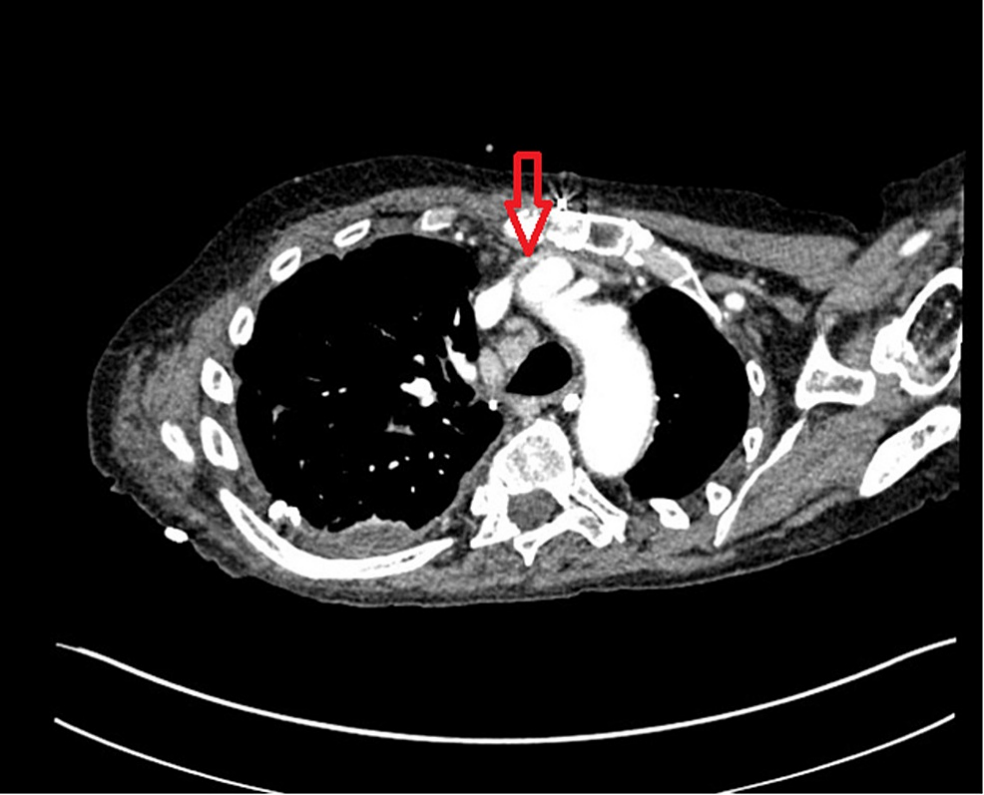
Chest CT scan with contrast done the day of catheter placement showing severe narrowing of the left brachiocephalic vein. CT: computed tomography
